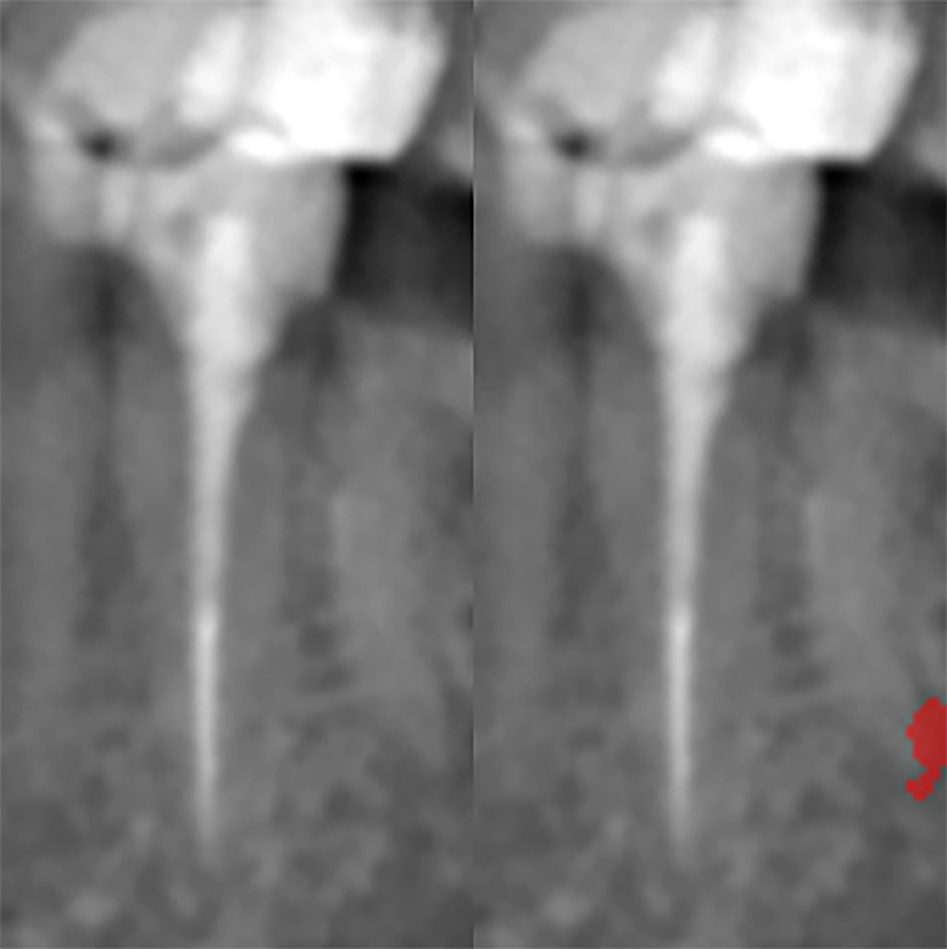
Incorrect periapical lesion localization mask at tooth 45. The lesion was predicted at the adjacent tooth 46.
